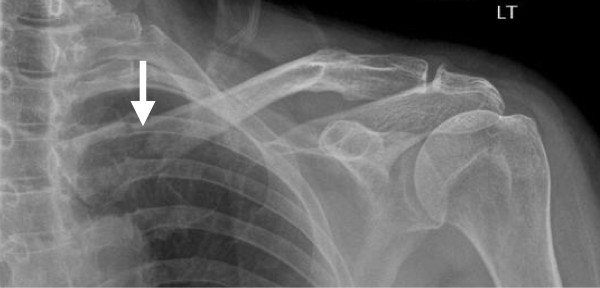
Plain radiograph of the left shoulder at the first presentation. The radiograph demonstrates a medial clavicular fracture (arrow) that was later diagnosed as pathological.
